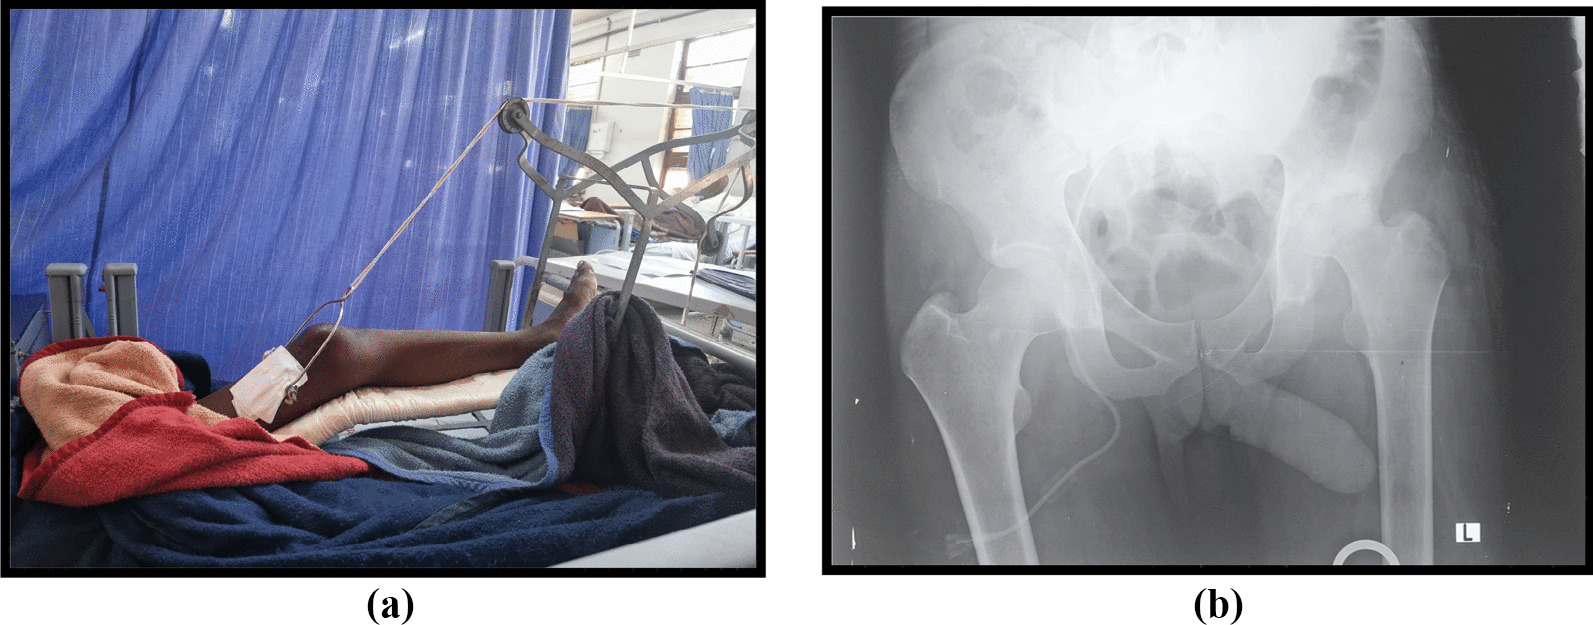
a 4 weeks on skeletal traction. b post-traction radiograph.
